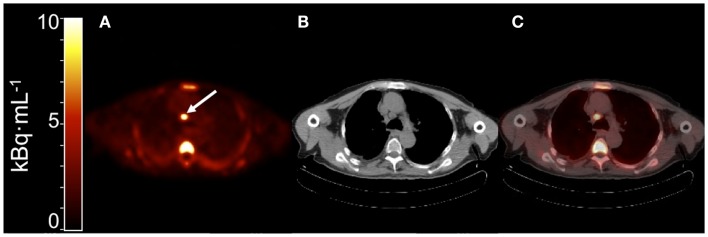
(A) Summed PET image of [^11^C]docetaxel uptake from 10 to 60 min post injection showing a mediastinal metastasis with increased uptake [arrow; (12)]. (B) Corresponding CT image. (C) PET-CT fusion image.
